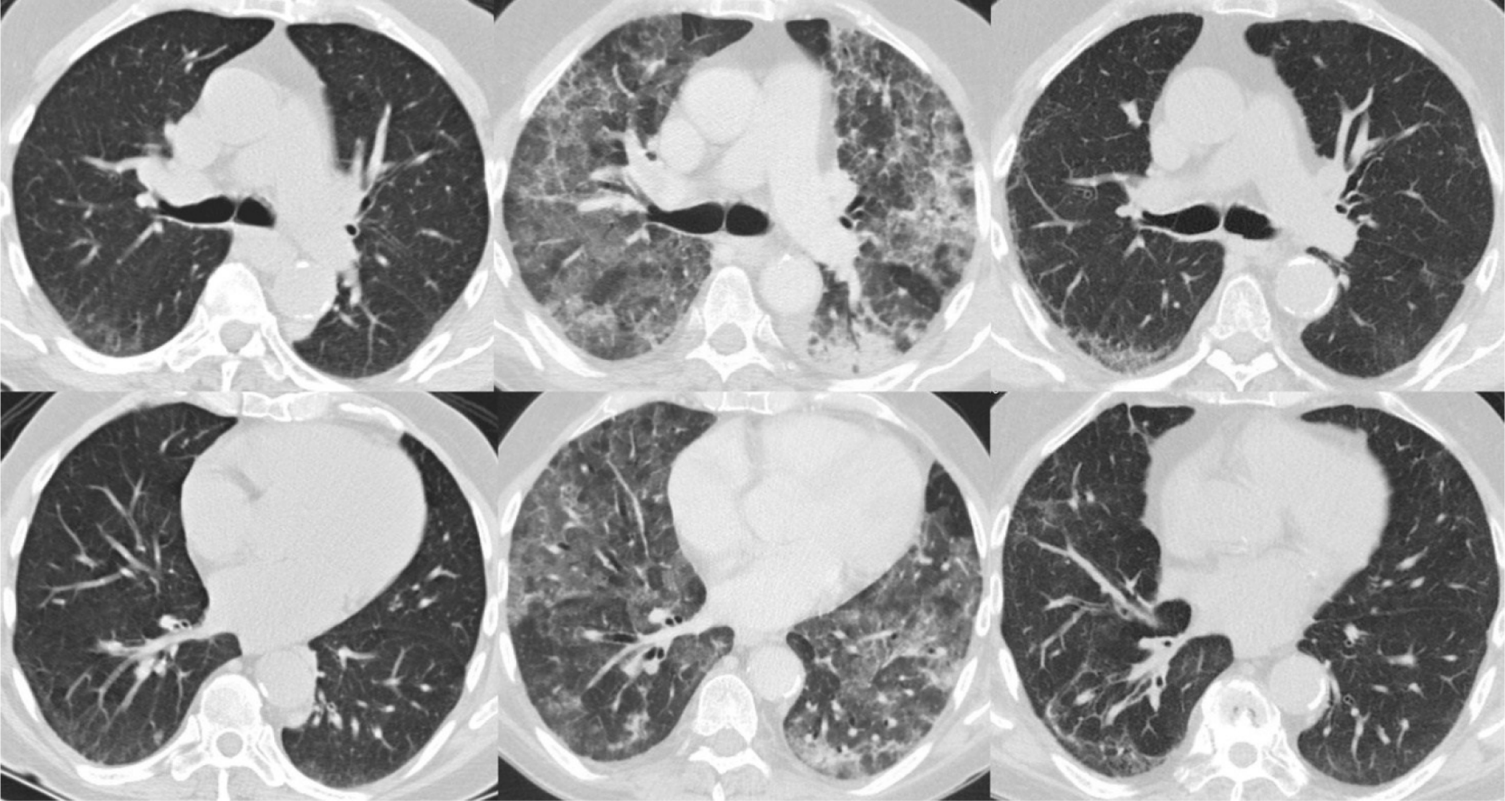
CT of the lungs at the level of carina and right inferior pulmonary vein prior to treatment with rituximab (left panel), during treatment with rituximab (middle panel), and after discontinuation of rituximab and initiation of steroid treatment.
